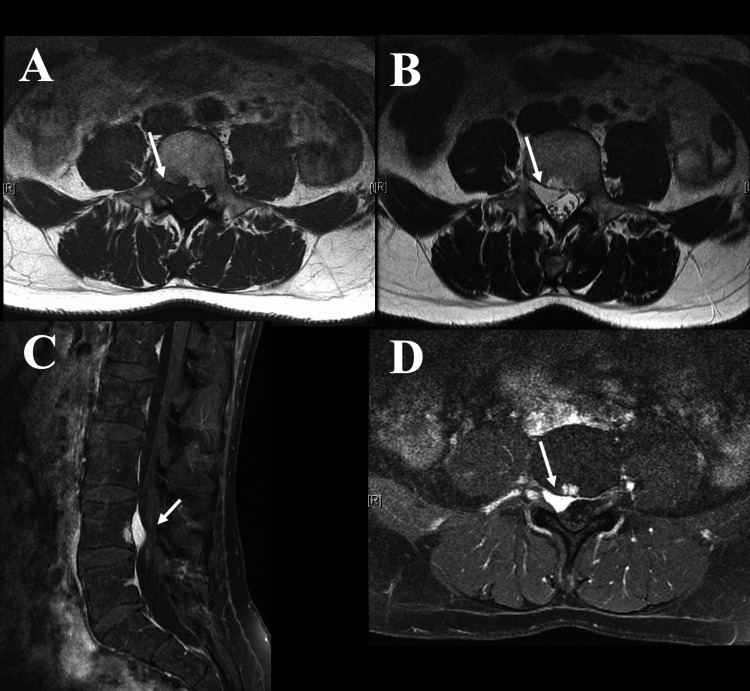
Preoperative MRI evaluation. Preoperative axial T1-weighted (A) and axial T2-weighted (B) MRI scans showing an extradural lesion compressing the nerve root L4. Contrast-enhanced T1 weighted sagittal (C) and axial (D) MRI scans demonstrates homogenous enhancement of the lesion. As differential diagnosis other pathologies such as lymphoma, leucaemic infiltration, plasmocytoma, and metastasis of an unknown primary tumor were considered.
